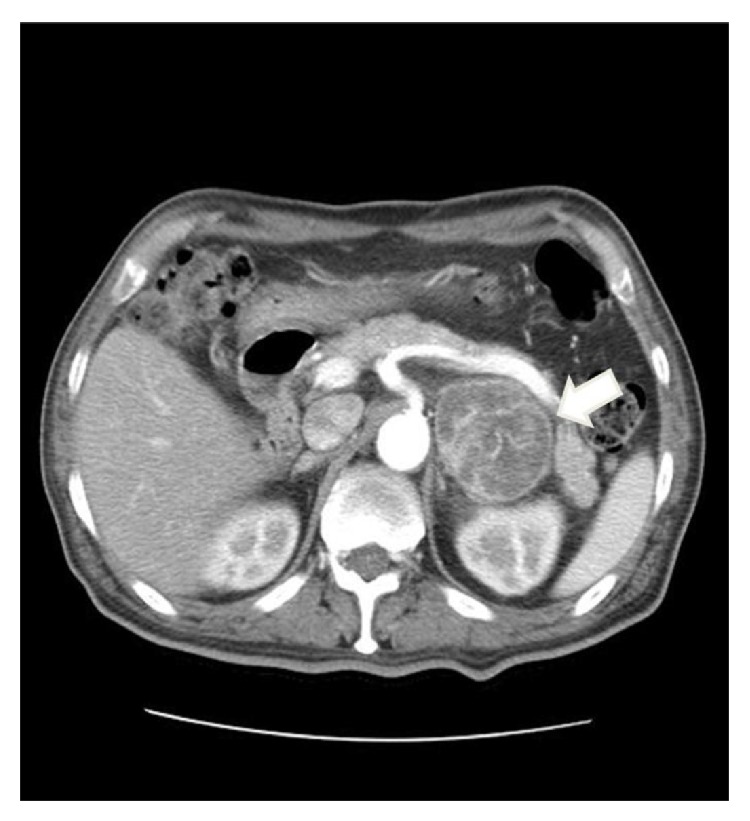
Contrast-enhanced abdominal computed tomography shows a heterogeneously enhancing left adrenal mass (4.8 × 5.7 cm) with irregular internal tumor necrosis (white arrow). There was no abnormality in the right adrenal gland (not shown).
